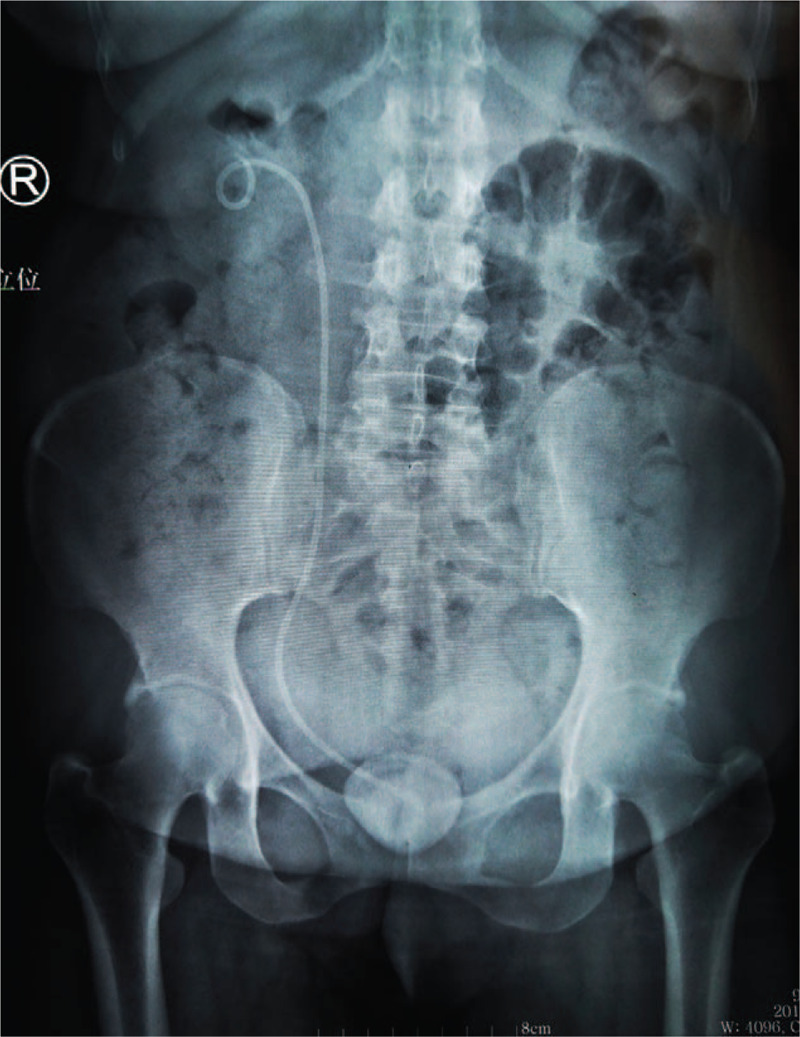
Preoperative KUB x-ray showed an entire coiled D-J stent with a giant stone in the bladder. D-J stent = double-J stent, KUB = kidney ureter bladder.
